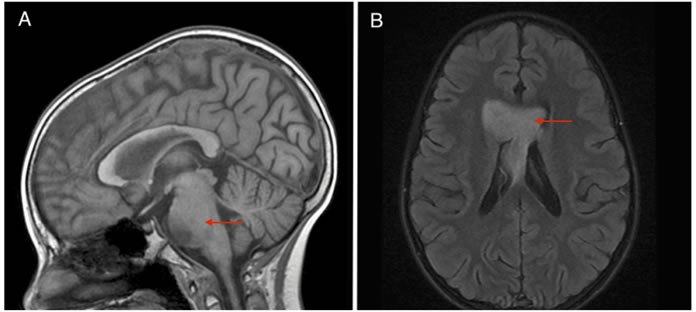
A. Sagittal SPGR of brainstem tumor progression and B. Axial T2 FLAIR contrasted images of concomitant metastatic lesion growth
